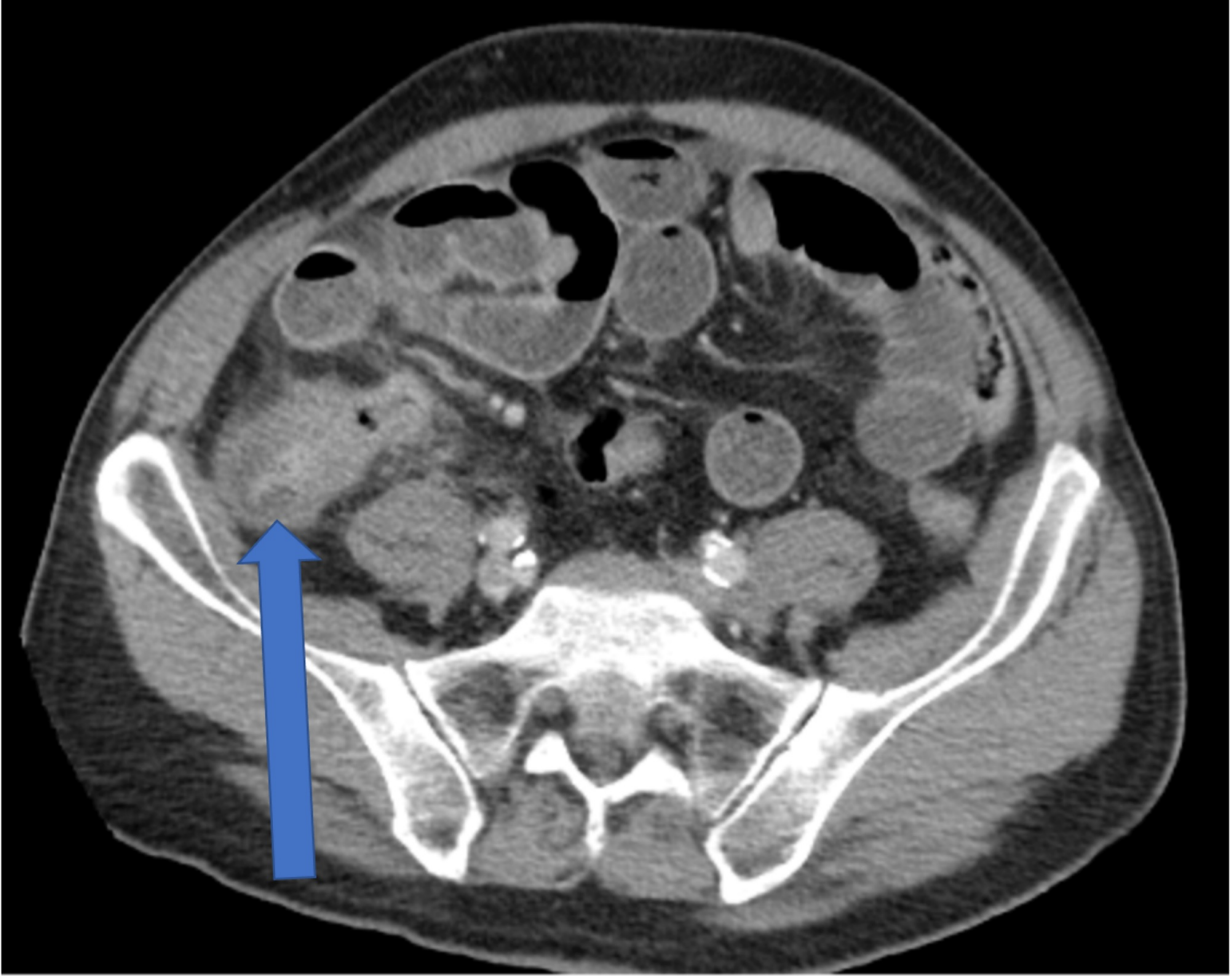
CT abdomen/pelvis on the second admission Ileocecal mass (arrow) causing obstruction
